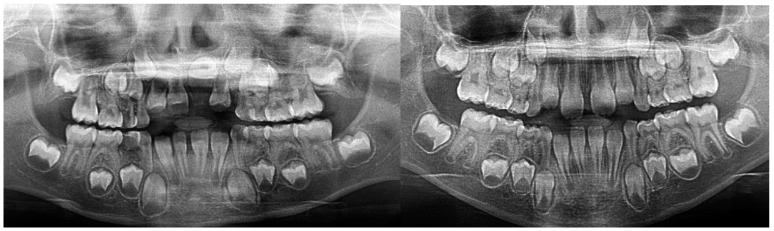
Panoramic radiography images taken during the first visit of the patient and her twin sister in February 2022.
